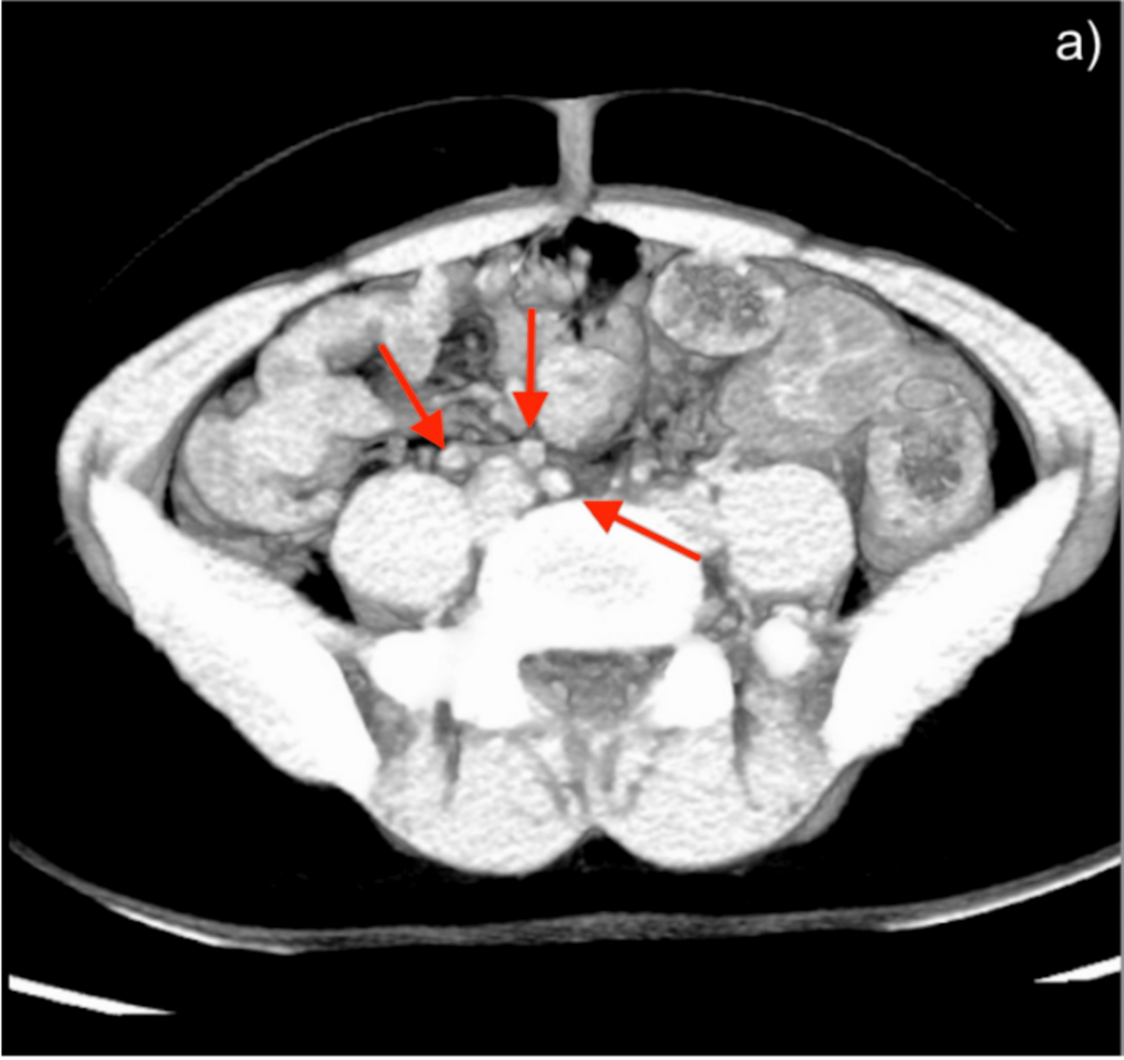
Axial abdominal CT scan. (a) Findings consistent with mesenteric adenitis. Multiple oval-shaped images correspond to swollen mesenteric lymph nodes. CT: computed tomography
